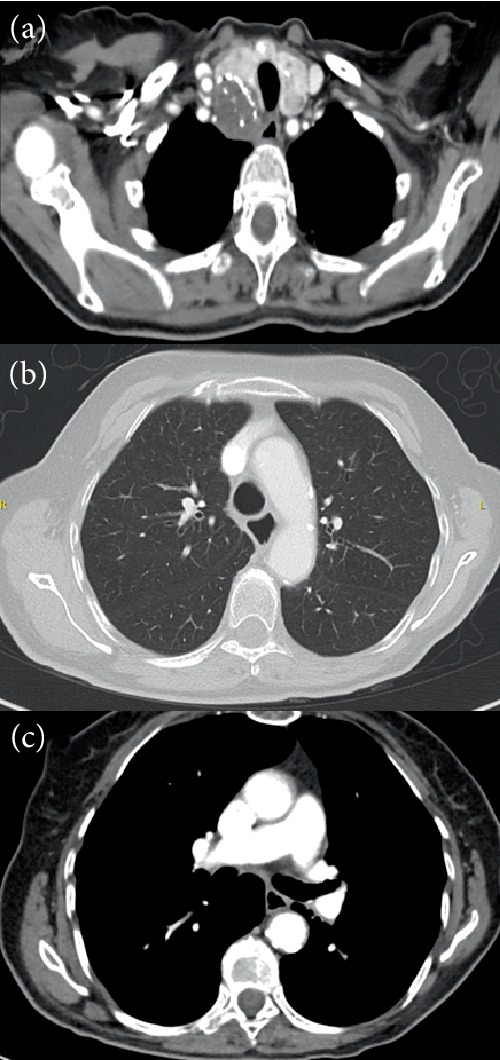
Chest CT 6 months after start of pembrolizumab showing ongoing response.
